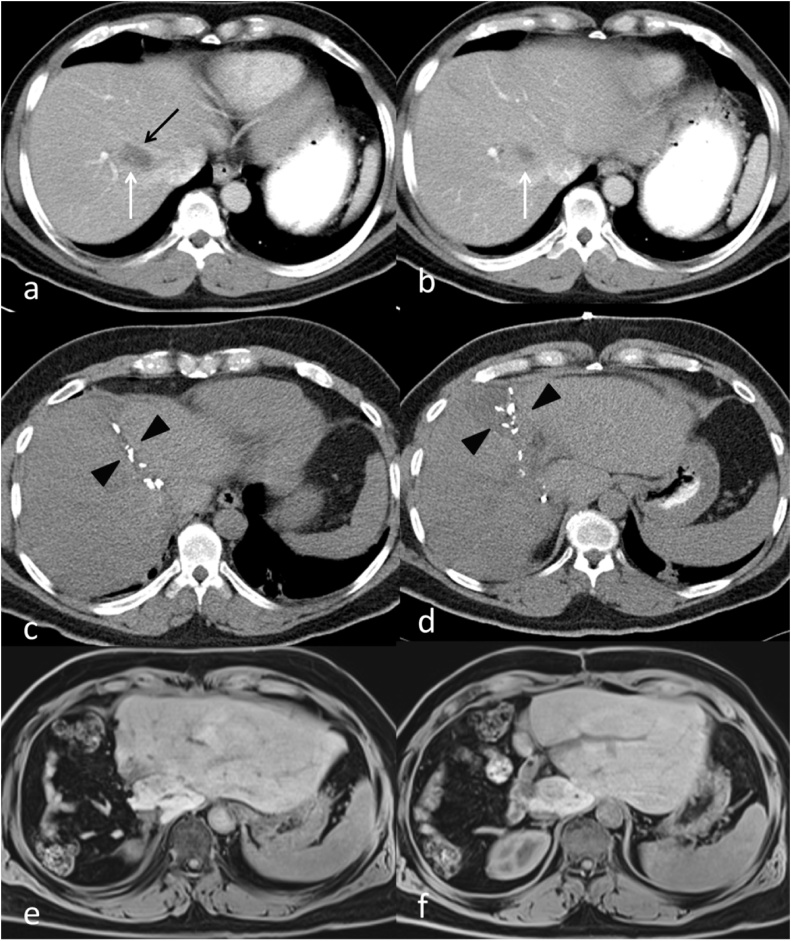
Patient 2: Preoperative contrast-enhanced CT (a,b) shows liver metastasis of the right lobe of the liver (white arrows), intimately related to the middle hepatic vein (black arrow). Post-stage-1 unenhanced CT on postoperative day 6 (c,d) shows the liver partition plane metallic clips (black arrowheads). Post-stage-2 unenhanced T1-weighted MRI at 6 months post stage 2 (e,f) shows obvious increase in size of the remaining liver.
